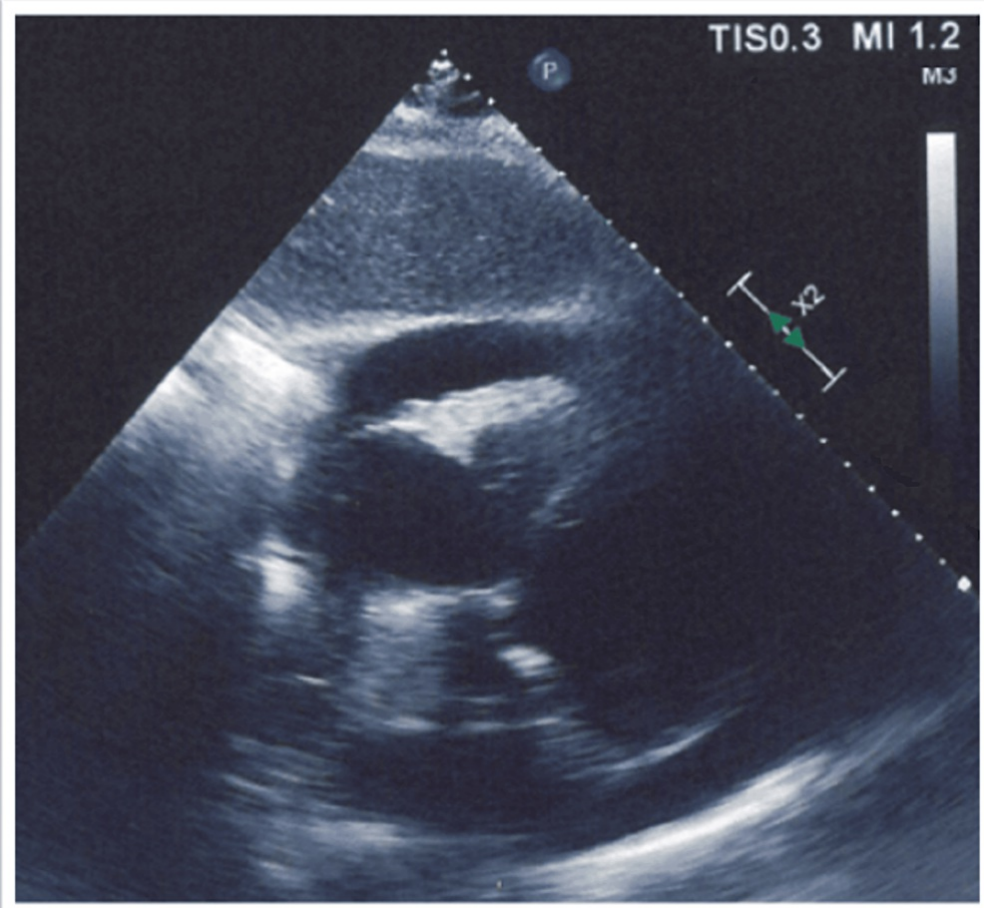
Transthoracic echocardiography revealing a moderate-large pericardial effusion.
